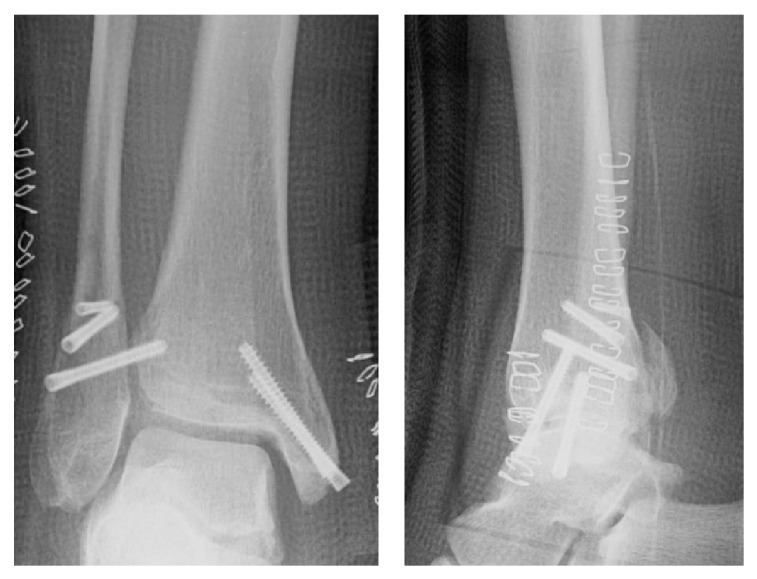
Radiographs soon after the 1st operation. Medial and lateral malleolus were fixed by Acutrak 2 screws.
